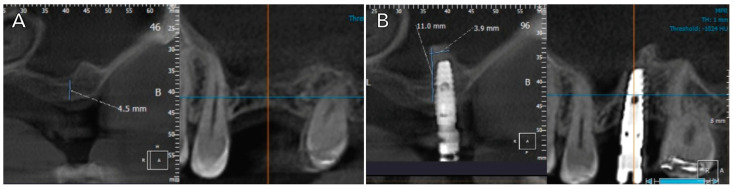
(A) Preoperative CBCT; (B) post-operative CBCT (5-month follow-up before impression taking).
